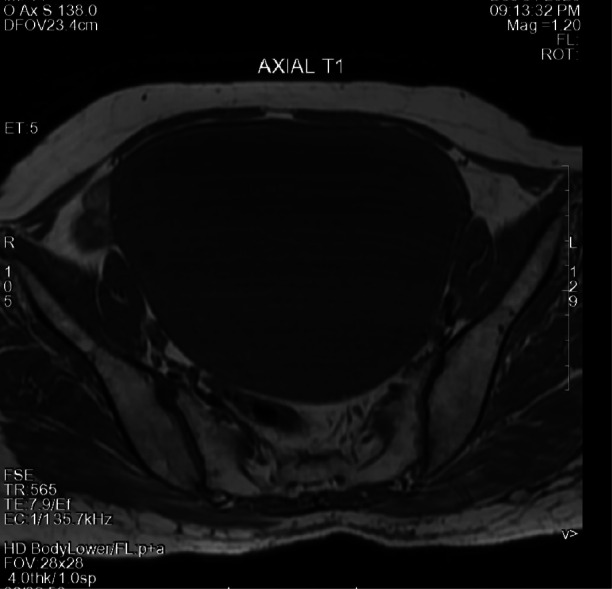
MRI axial view of the same
